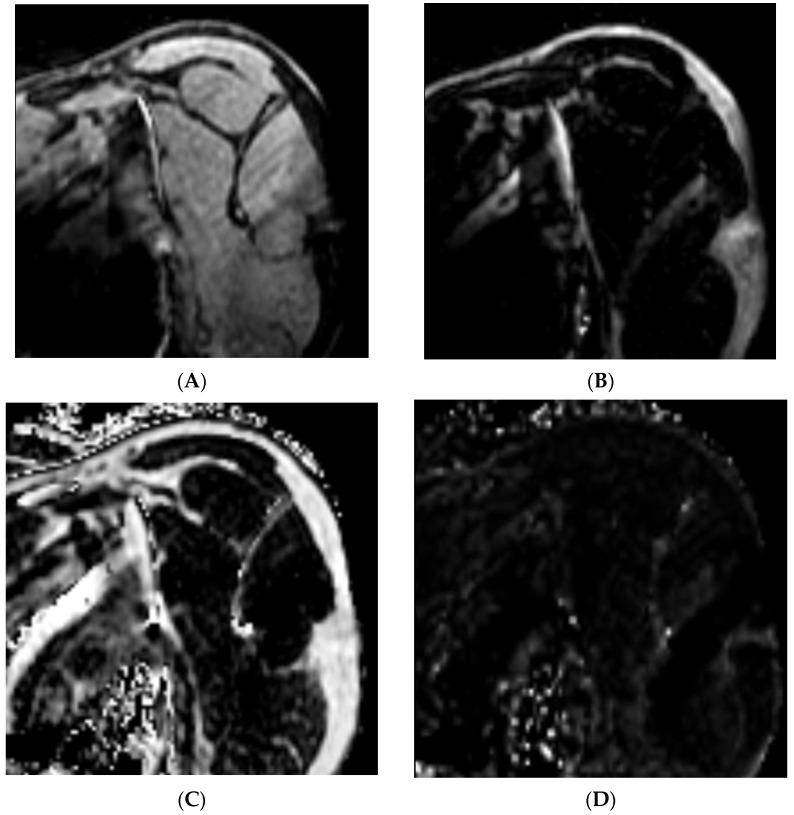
Dixon sequencing generates four unique data sets of the same MR image: (A) water only, (B) fat only, (C) in phase, and (D) opposed phase.
